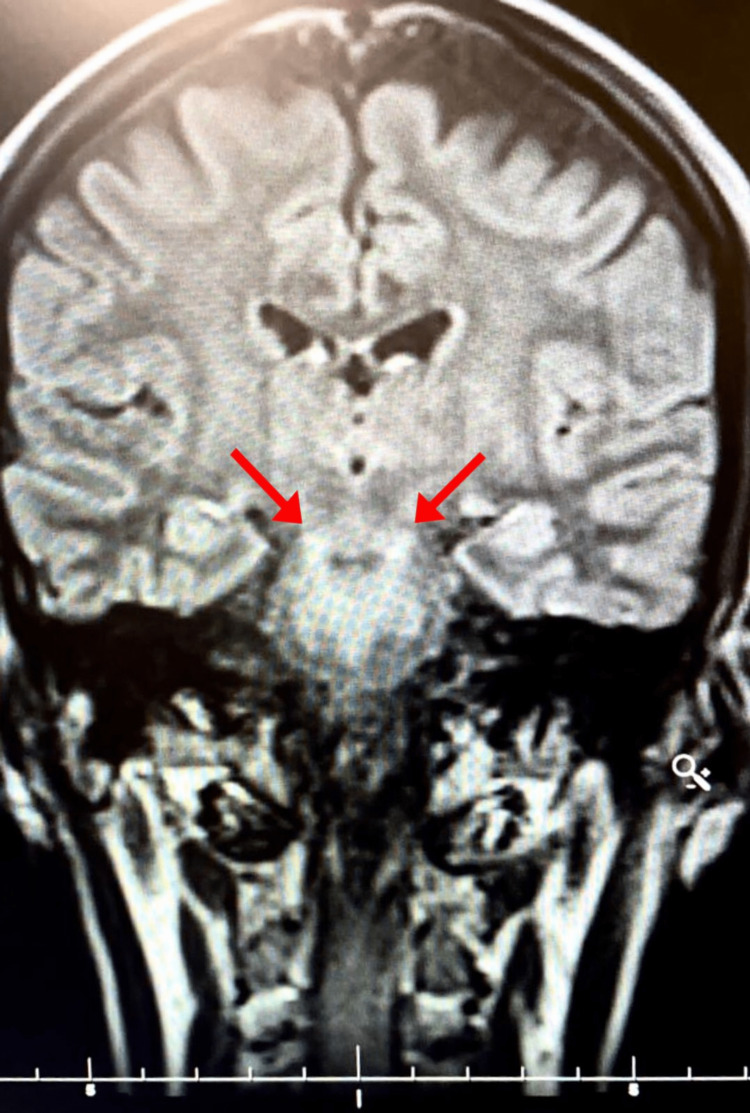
T2-weighted MRI showing pontine hyperintensities (arrows)
